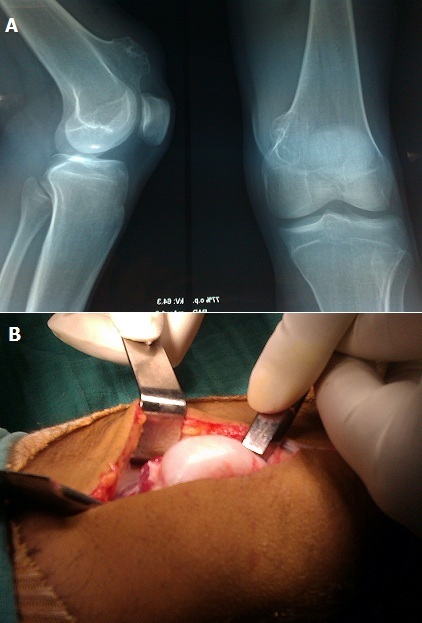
A) radiographie du genou droit de face et de profil montrant une exostose de l′extrémité inférieure du fémur sessile; B) image per opératoire montrant l′exostose géante
